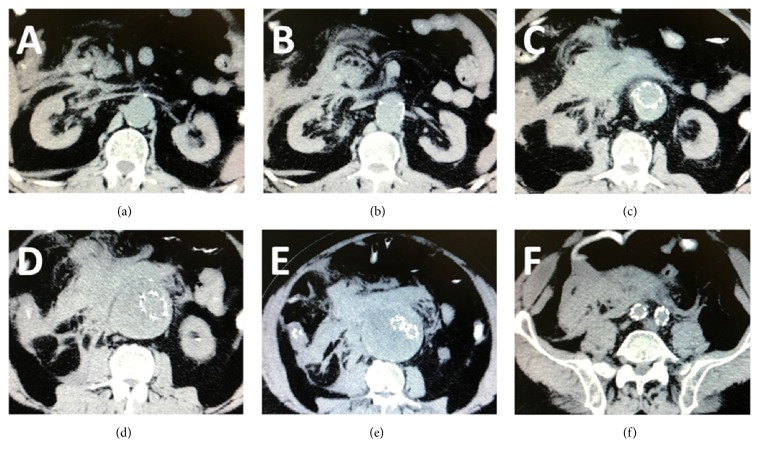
Preoperative CT scan. Contrast media had to be avoided due to severe renal failure. The Talent stent-graft was migrated and the proximal sealing was lost ((a)–(c)) leading to potential type I endoleak, sac enlargement, and rupture. A leaking 9 cm AAA with a huge retroperitoneal hematoma was depicted ((d) and (e)). Distal iliac sealing was sufficient (f).
